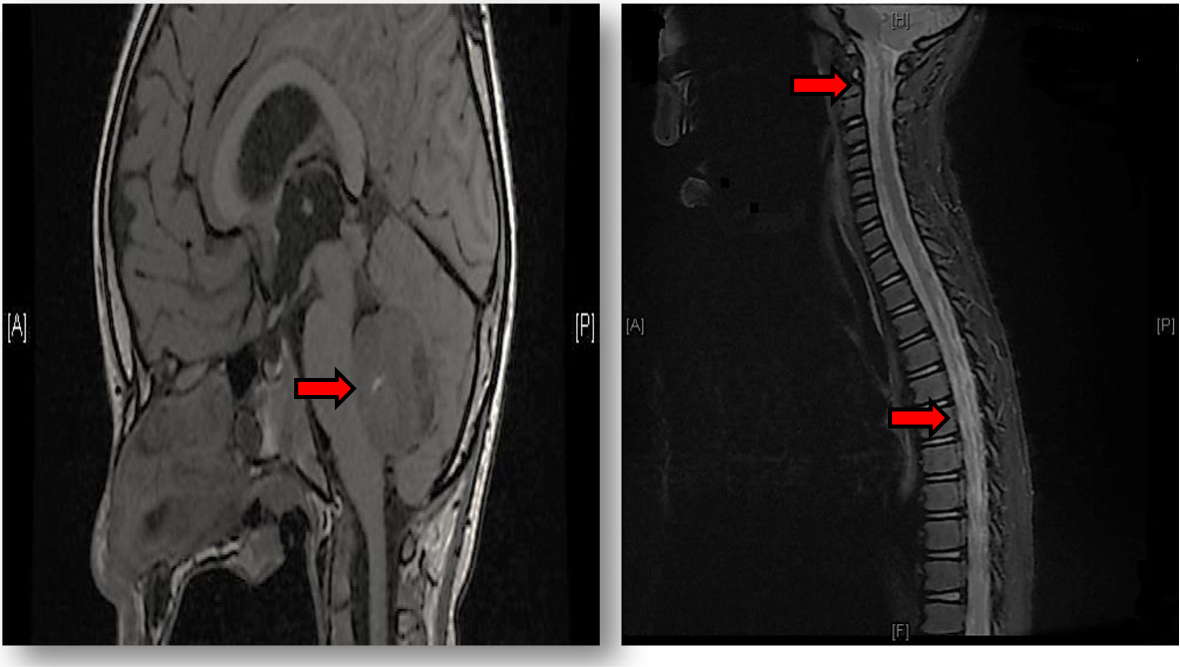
Brain and spine MRI showed a midline posterior fossa tumor suggestive of medulloblastoma with leptomeningeal enhancement concerning for intraspinal seeding (arrows)
